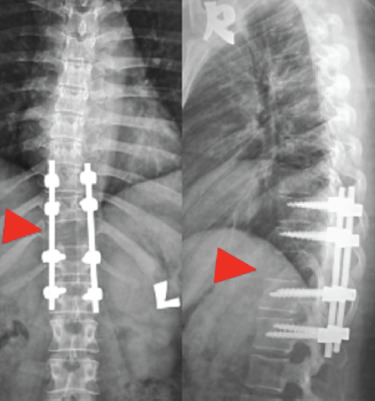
Spinal radiograph eight months after surgery demonstrates T11 vertebrae body radiolucency (arrowhead). Implants are intact, with no other vertebrae involvement.
